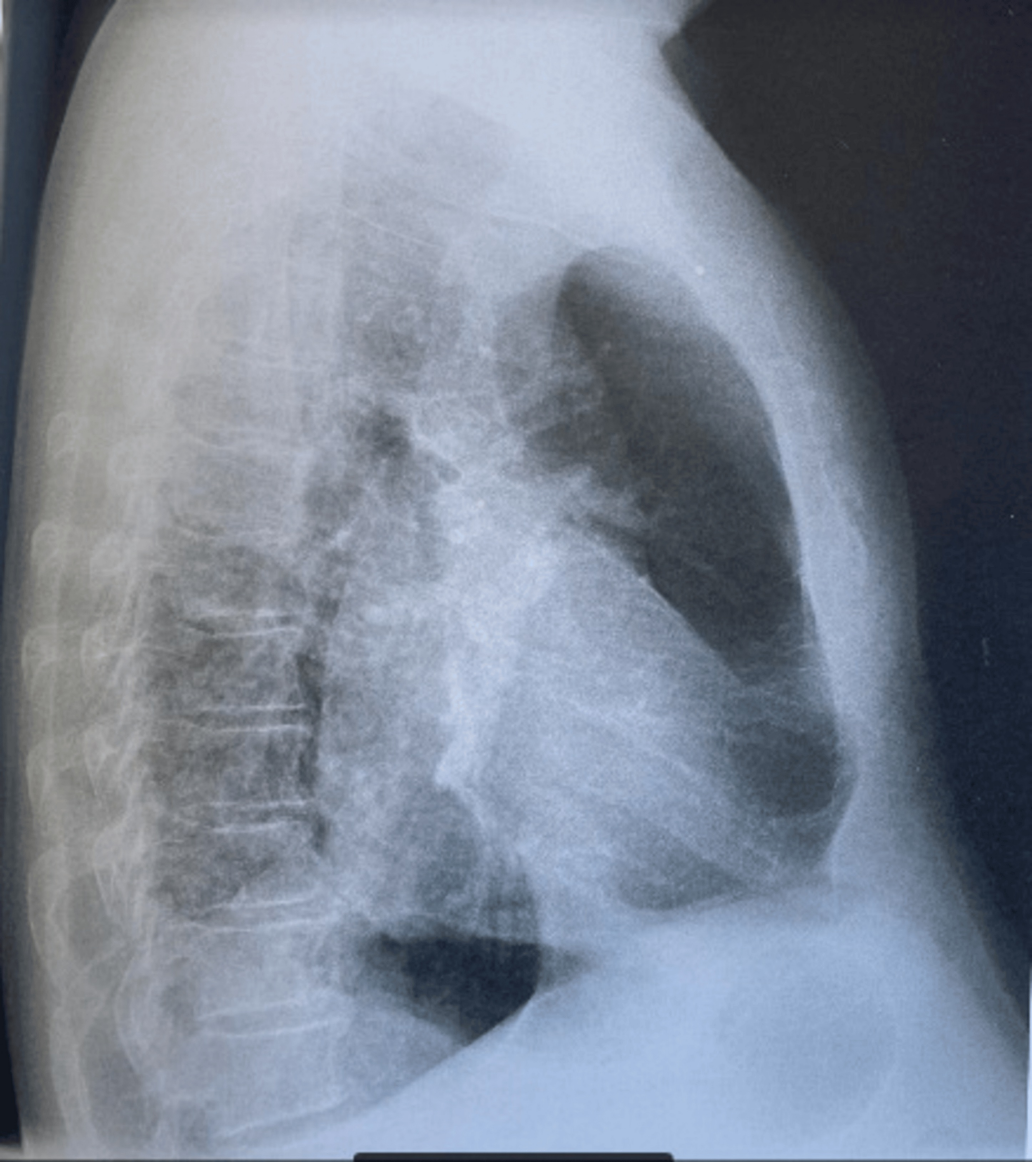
Lateral chest X-ray Left basal lung opacity that obliterates the edge of the heart, associated with atelectasis and a small left lung field
